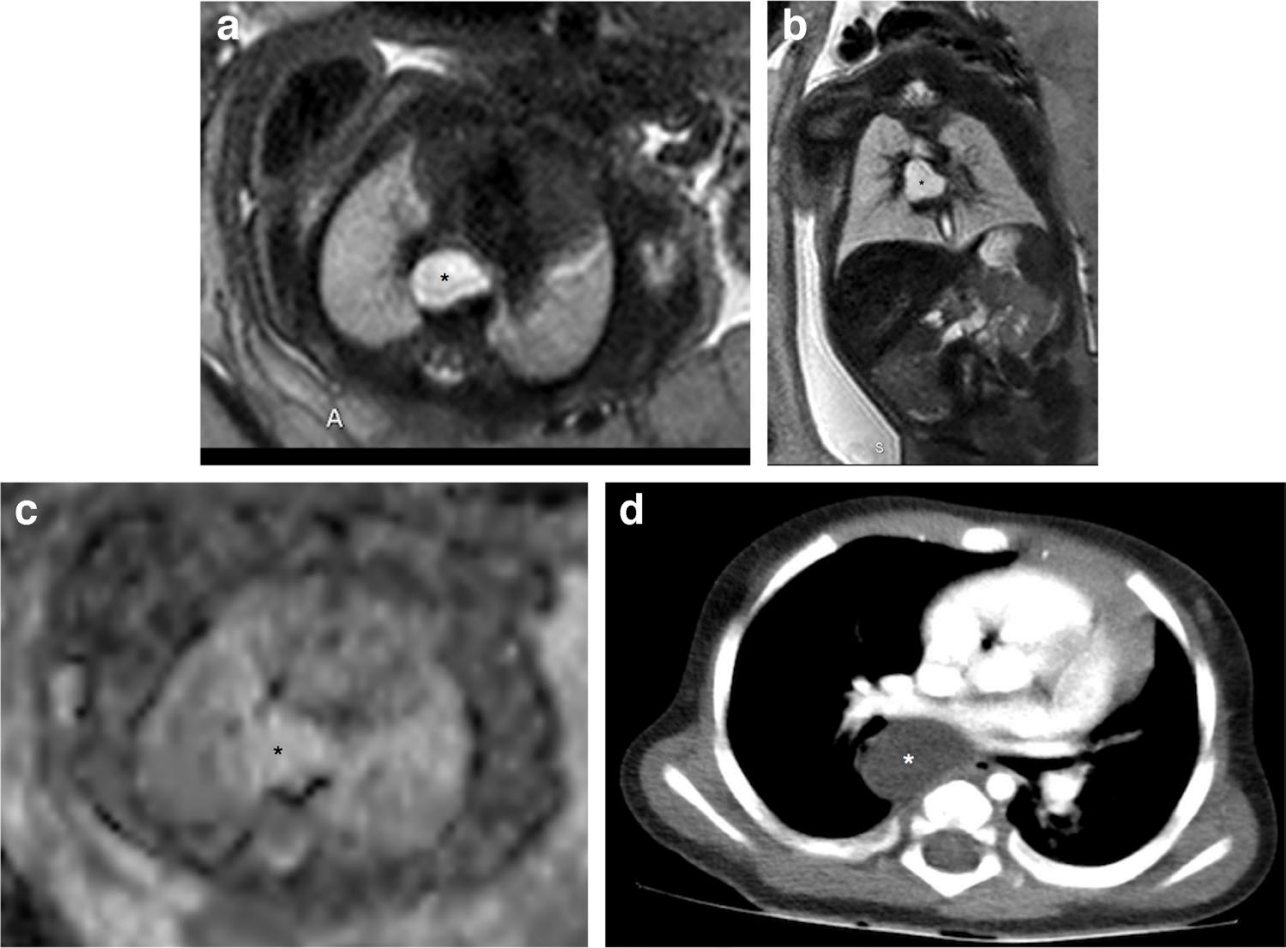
A bronchogenic cyst in a 27-week gestational age female fetus. a–c Single-shot T2 axial (a) and coronal (b) and axial apparent diffusion coefficient (ADC) (c) magnetic resonance images show a well-circumscribed homogeneous lesion (*asterisks*) in the right perihilar region, posterior to the right pulmonary veins and below the carina. It shows homogeneous high signal intensity on T2 and ADC map in keeping with a cystic lesion with clear contents, consistent with a bronchogenic cyst. d A post-contrast axial computed tomography image obtained at age 7 months confirms a homogeneously hypoattenuating cystic lesion in the mediastinum, posterior to the right pulmonary veins and left atrium and below the carina (*asterisk*). Surgery confirmed a bronchogenic cyst
